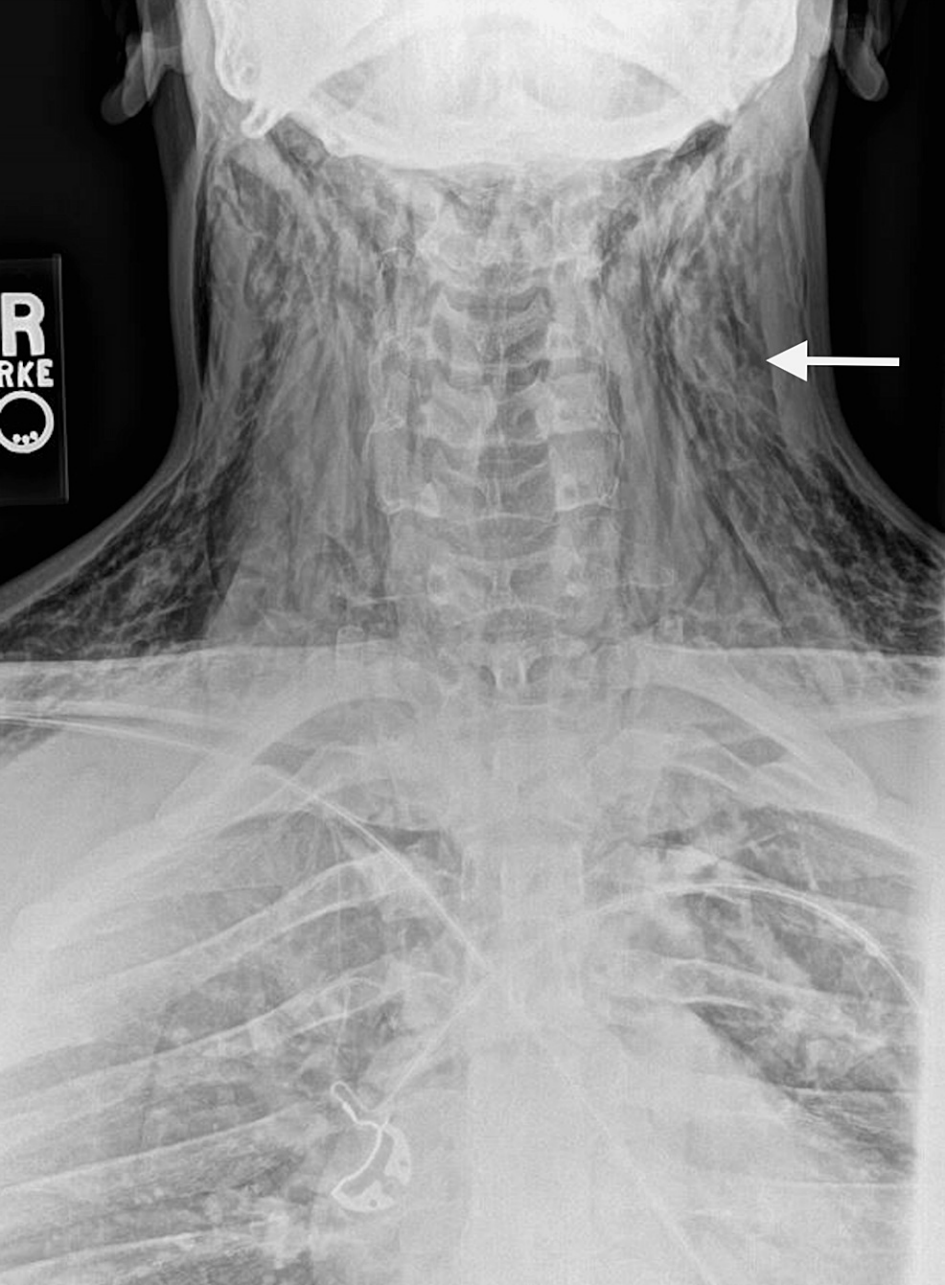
Neck radiograph with an arrow pointing to subcutaneous air
